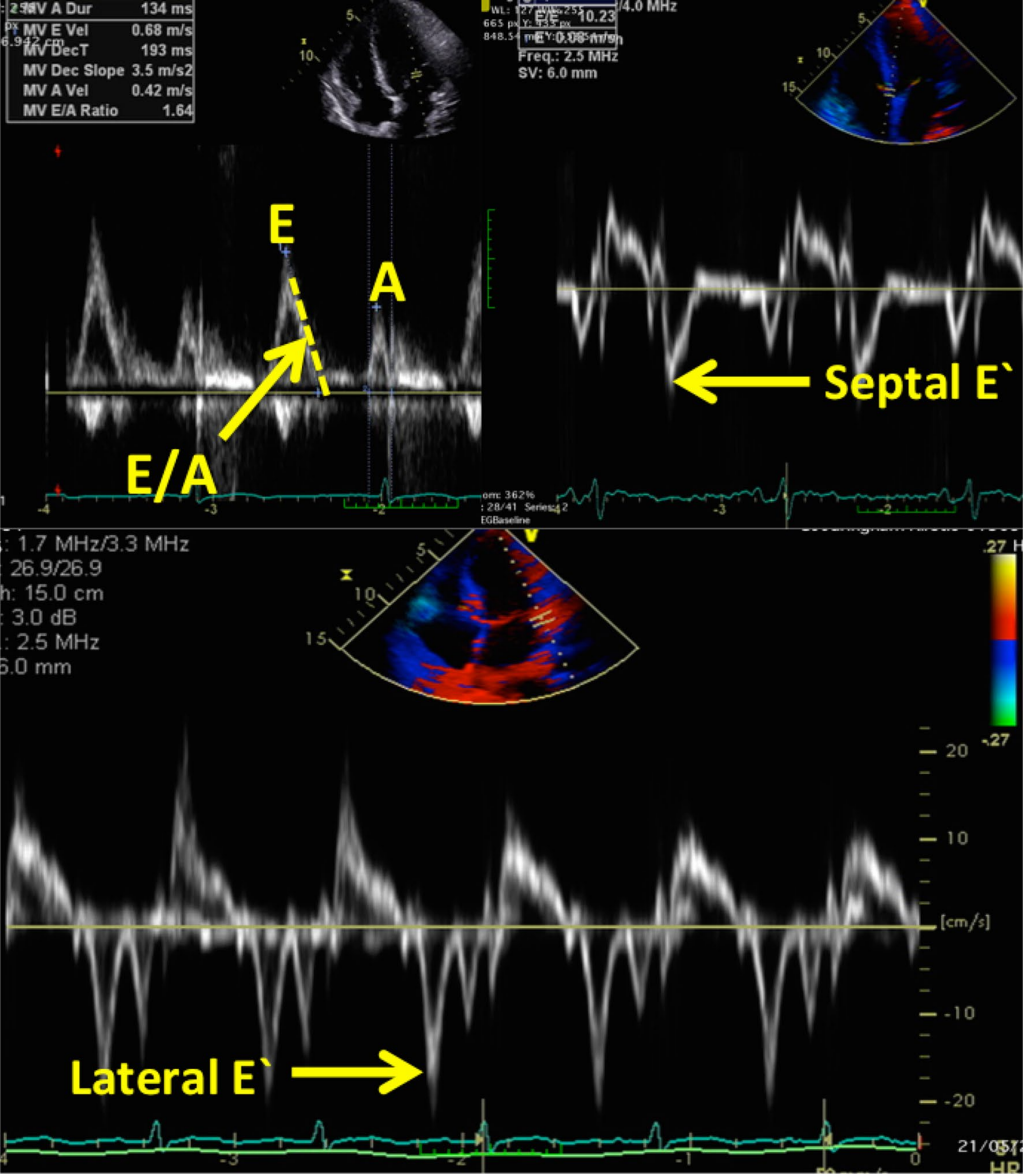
Examples of Doppler assessments used for assessment of left ventricular diastolic function (trans-mitral inflow and tissue Doppler assessment of the septal and lateral mitral valve annulus). *A*, late diastolic trans-mitral flow velocity wave, *E* early diastolic trans-mitral flow velocity wave, *E′*, early diastolic mitral annulus velocity
